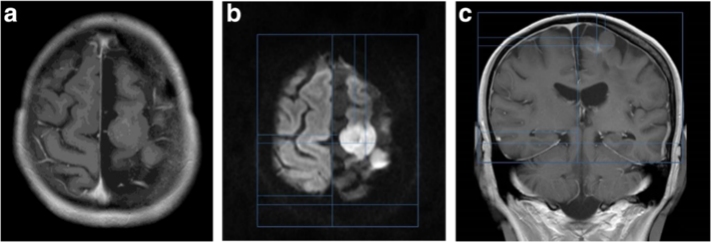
a, b & c depict treatment planning in a patient with recurrent anaplastic astrocytoma incorporating DWI sequences. Figure a shows an axial T1 postcontrast slice demonstrating punctate enhancement. Head size measurements were performed in a standard manner on T1 sequences (not shown). Figure b shows the axial DWI sequence with a clear area of hyperintense signal in the region of active disease which was used to obtain axial tumor location measurements. A reference frame is approximated around the head at the level of the scalp. Tumor location coordinates are measured from the right frame to the edges of the DWI signal abnormality thought to represent active tumor at the discretion of the treating physician. Figure 8c shows the coronal T1 postcontrast slice used to map tumor coordinates in the coronal plane. Treatment was planned measuring from a reference frame drawn at the level of the scalp extending to the lowest margin of visible tentorium. Tumor location coordinates are measured from the right frame to the edges of faint enhancement and gyral thickening
